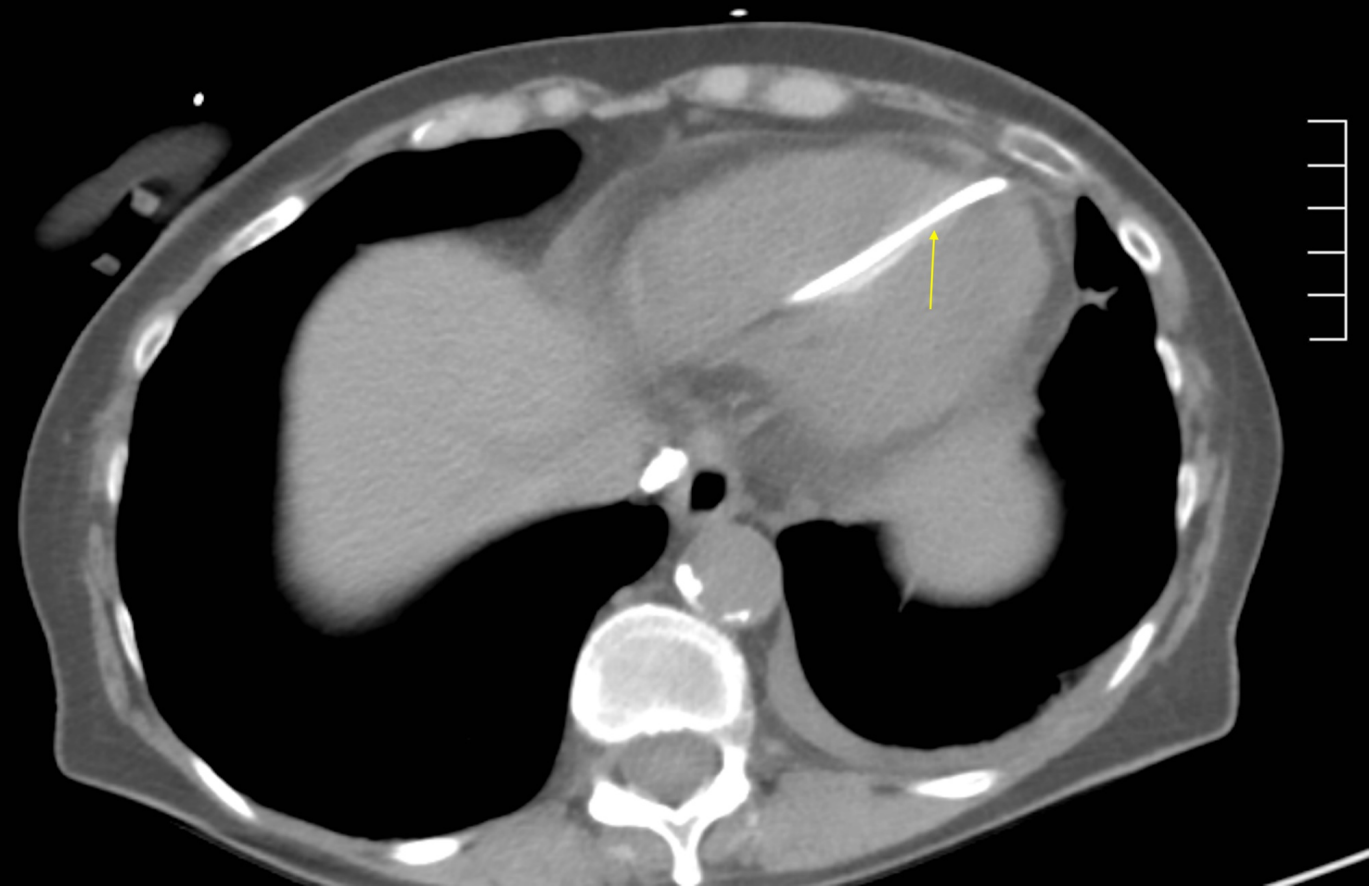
CT chest without contrast This image is a coronal view of a chest CT showing the pacemaker lead penetrating through the right ventricle and pericardium.
